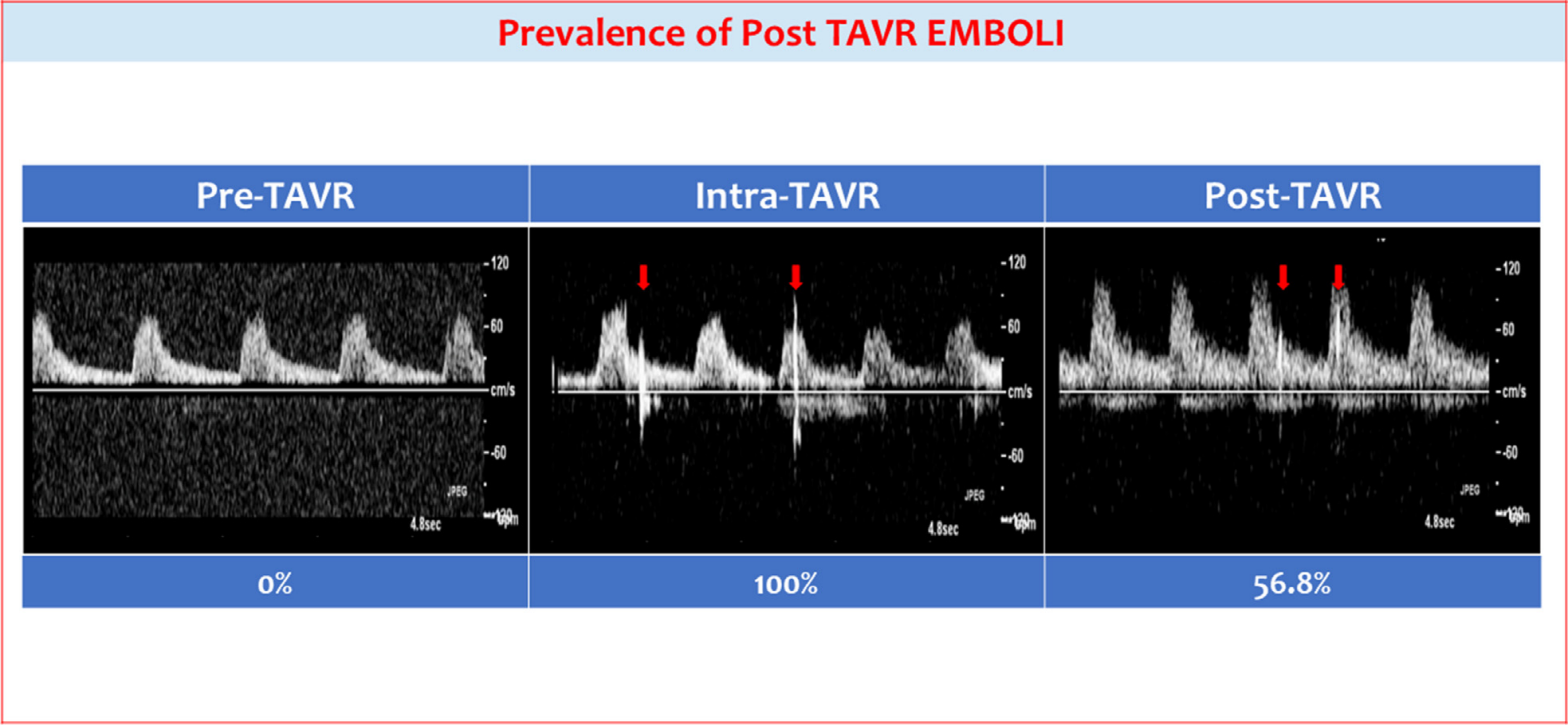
No patients had pre-transcatheter aortic valve replacement high-intensity transient signals (HITS). All patients with intra-TAVR transcranial Doppler had evidence of HITS. Of the patients, 56.8% continued to have HITS at 24 hours post-TAVR.
